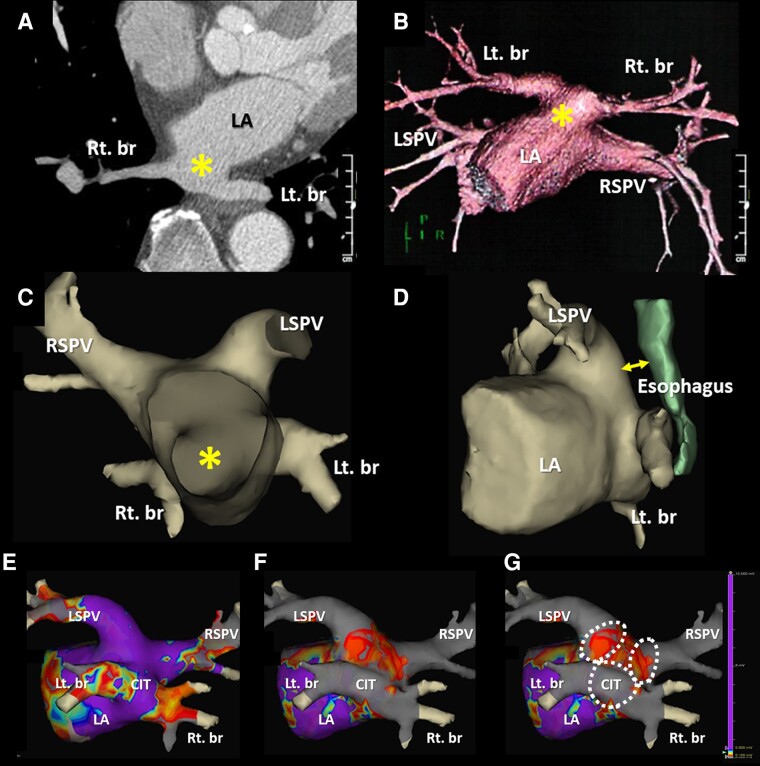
A computed tomography projection in the oblique axial view presents the inferior pulmonary veins entering the left atrium via a common ostium (asterisk in *A*). A volume-rendered three-dimensional computed tomography image of the common ostium of the right and left inferior pulmonary veins (*B*) and the endoscopic view from the front (*C*) and the left (*D*) demonstrate the presence of a large common inferior trunk of the pulmonary veins arising from the posterior wall of the left atrium. The EnSite™ images viewed from the back before (*E*) and after (*F* and *G*) ablation using a third-generation (X3) laser balloon for the three pulmonary veins. The white dotted lines represent the ablation lines of the X3-LB, which were deduced from the findings seen in *Figure 3E–H*. LSPV, left superior pulmonary vein; RSPV, right superior pulmonary vein; Lt. br, left branch; Rt. br, right branch.
